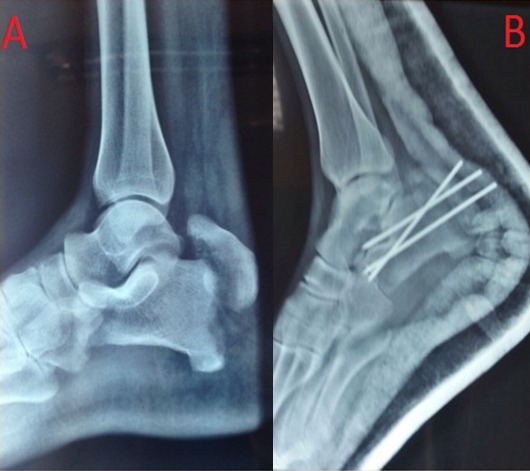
(A) radiographs of the right ankle demonstrating fracture of the tuberosity of the calcaneus; (B) X-ray on the first postoperative day
